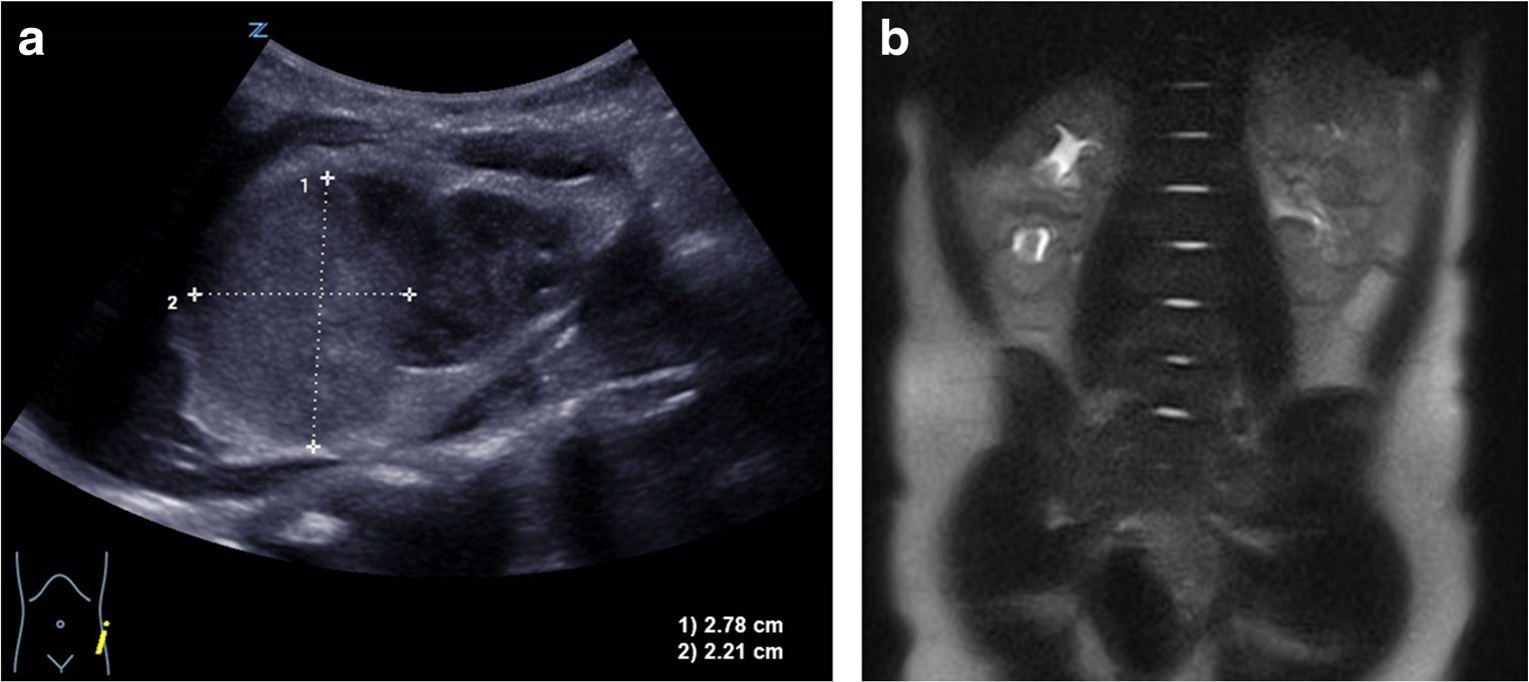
Postpartum (a) ultrasonography and (b) MRI. The studies show a solid tumor in the upper pole of the left kidney. The upper calyx group cannot be clearly delineated
